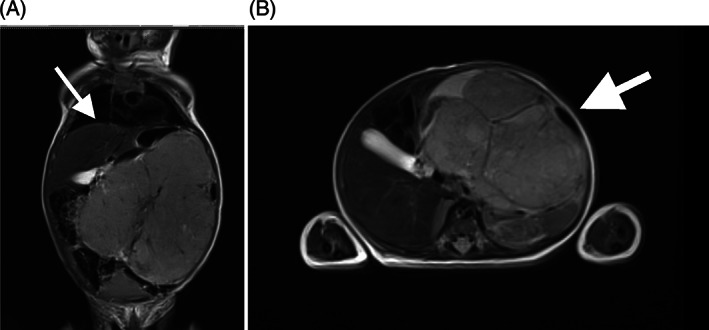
(A, B) Abdominal magnetic resonance image of an immature teratoma arising from mesenteric tissue in case 2. It should be noted that the mass compresses the intestines against the abdominal wall and significantly elevated the diaphragmatic domes, generating a compressive‐type alteration in respiratory mechanics (arrows)
